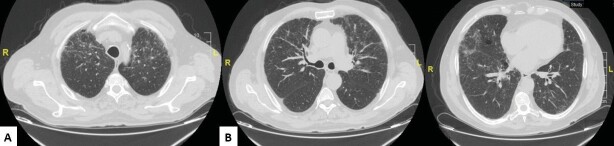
Figure 2. In chest computed tomography, irregular
